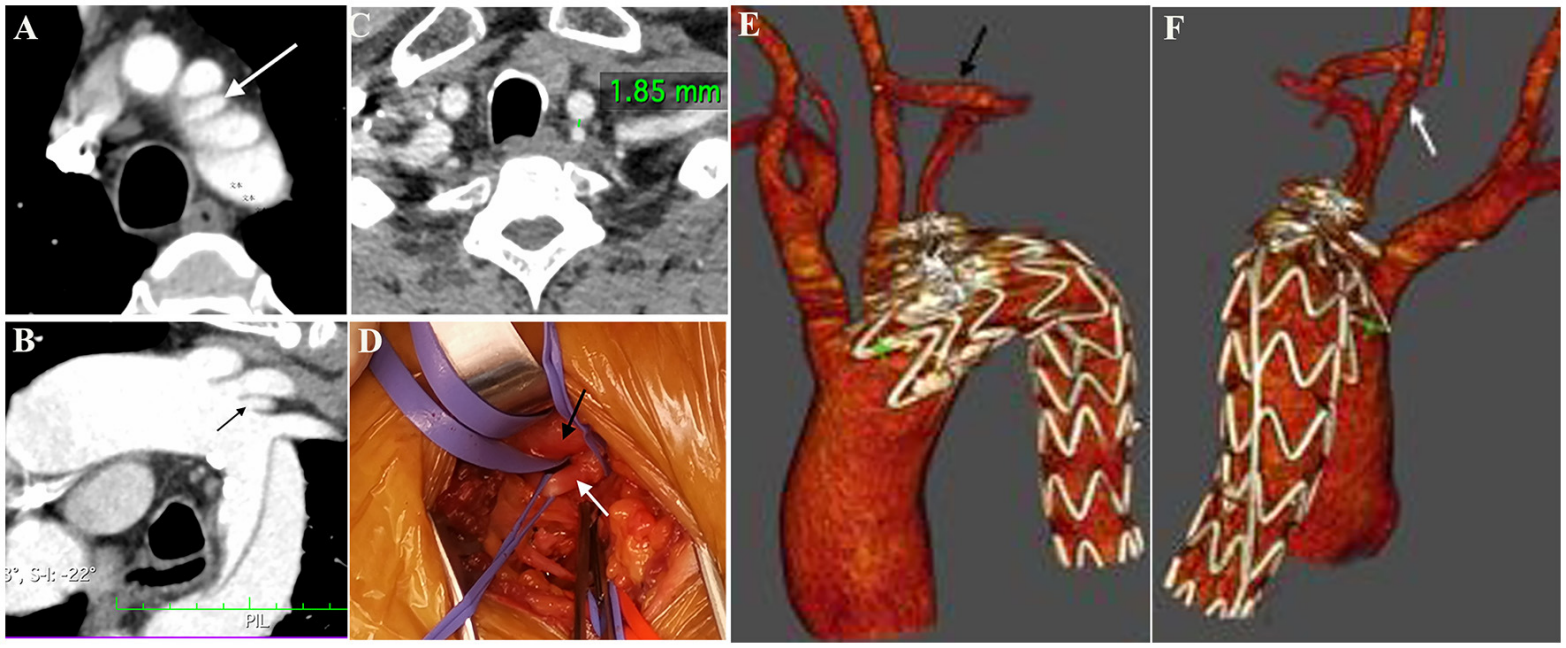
“Zone 2” TEVAR: Preoperative CTA showed the presence of a complex lesion of the descending aorta (A). Multiplanar reconstruction documented the presence of an ILVA [(B), white arrow] just proximal to the LSA origin. The distance between ILVA and LCCA was 1.85 mm (C). Intraoperative view (D) showing the anatomy of LCCA (black arrow) and ILVA (white arrow). A Follow-up CTA study with the 3D volume confirmed the patency of the CSbp (E) and the transposed ILVA (F). CTA, computed tomography angiography; LCCA, left common carotid artery; LSA, left subclavian artery; ILVA, isolated left vertebral artery; CSbp, carotid-subclavian bypass.
